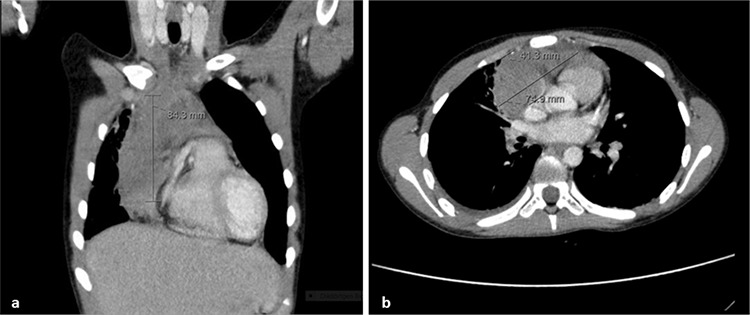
Computed tomography of the thorax showing anterior mediastinal mass in coronal (a) and axial (b) sections.
